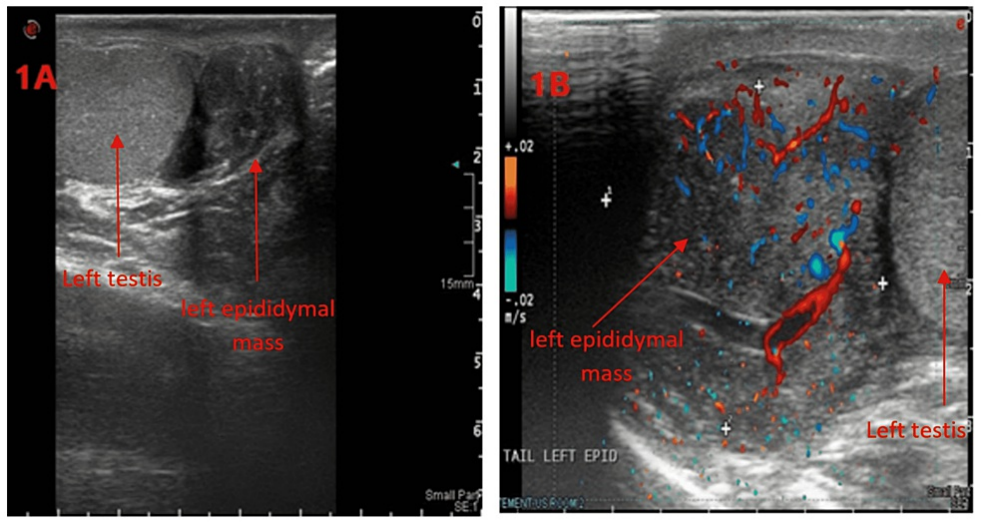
Testicular ultrasound (A) Ultrasound of the scrotum revealed left testis with multiple tiny parenchymal calcifications and left epididymal tail bulky mass contained multiple calcific foci as well. (B) The tail and body of the left epididymis were diffusely enlarged with hypoechoic echotexture of size (3.5×2.8 cm) with significantly increased vascularity with focal hypoechoic lesions with distinct margins, mostly consistent with inflammation.
